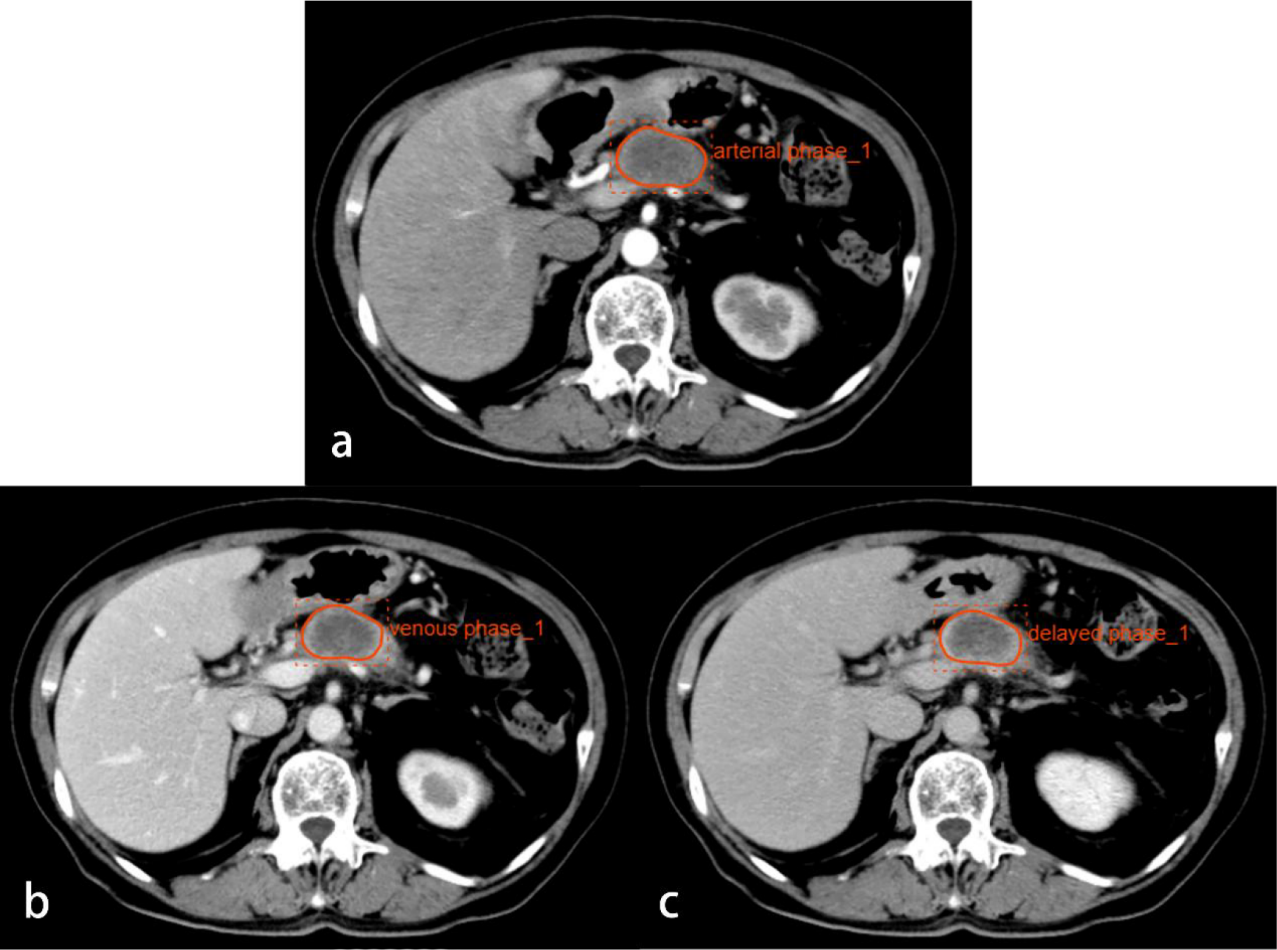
CT images from a 74-year-old woman with PDAC in the neck of the pancreas. Regions of interest are outlined in orange on arterial (A), venous (B), and delayed phase (C) images.
